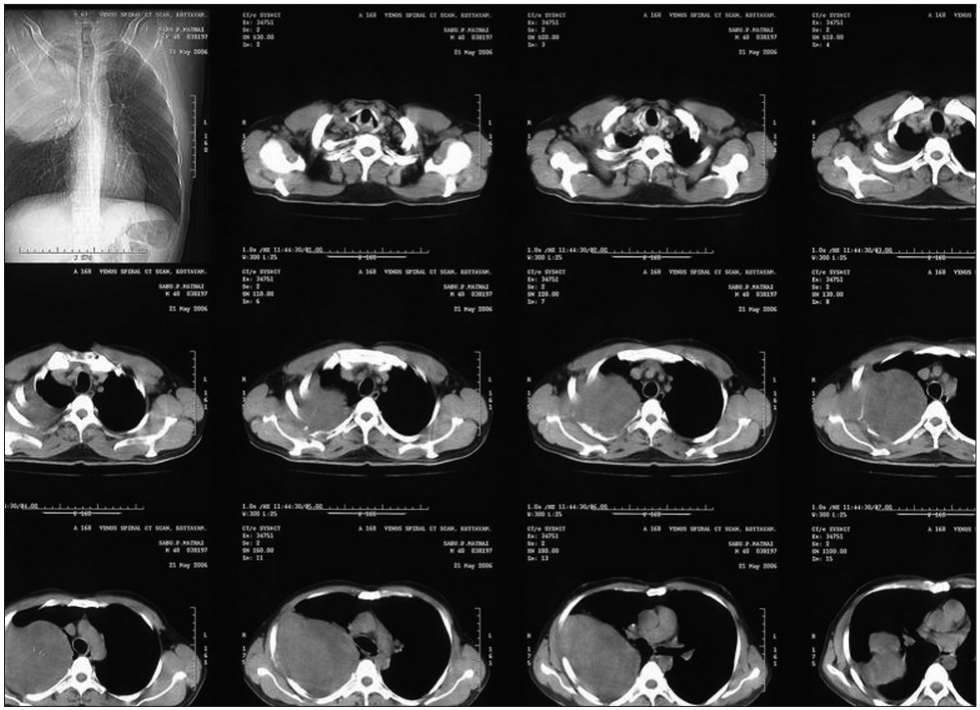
Spiral CT chest showing a large, well-defined soft tissue density mass lesion in the posterior aspect of the right upper hemithorax. The lesion is found to be extending to the chest wall with erosion of right upper ribs
